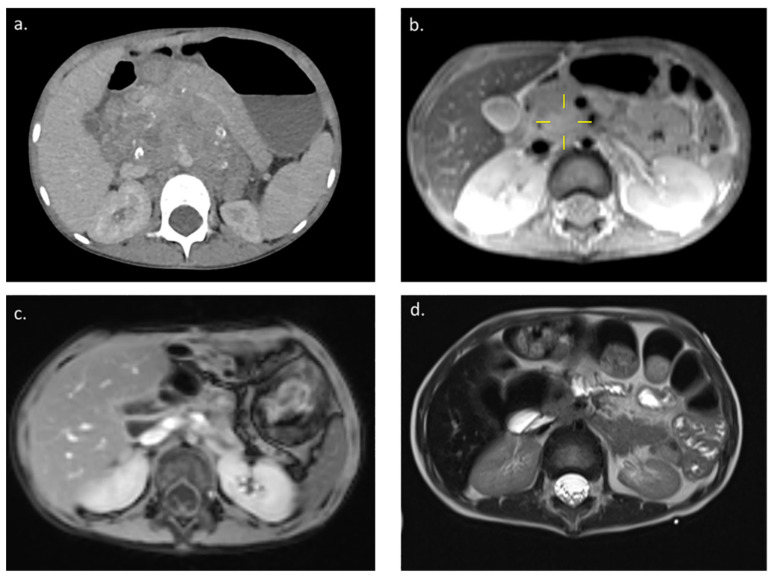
Contrast-enhanced computed tomography image of the abdomen of a girl, three years and six months of age, with high-risk neuroblastoma (a) at diagnosis, (b) prior to surgery (following rapid COJEC induction chemotherapy), (c) prior to receiving dinutuximab beta (after surgery, myeloablative chemotherapy, autologous stem cell transplant, and radiotherapy), and (d) after receiving dinutuximab beta. COJEC, cisplatin (C), vincristine (O), carboplatin (J), etoposide (E), and cyclophosphamide (C).
